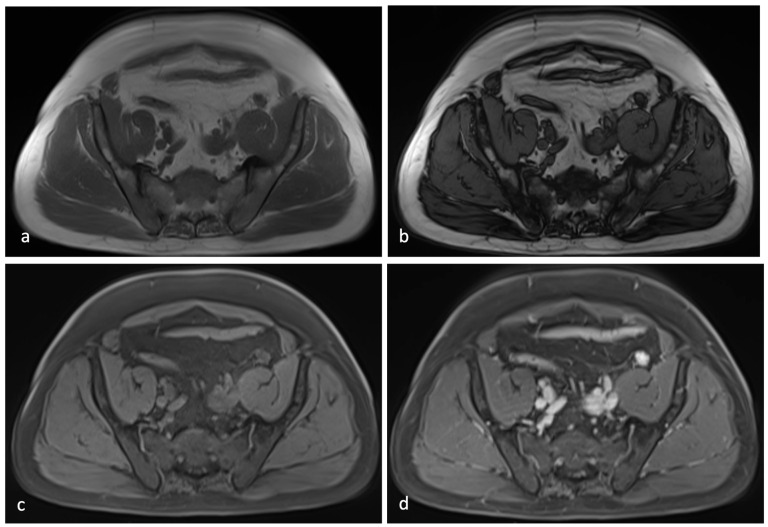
Axial DIXON T1-weighted sequences in (a) and out-of-phase (b). Pre-contrast T1w VIBE sequences (c) and post-contrast graphic sequences in late phase (d).
